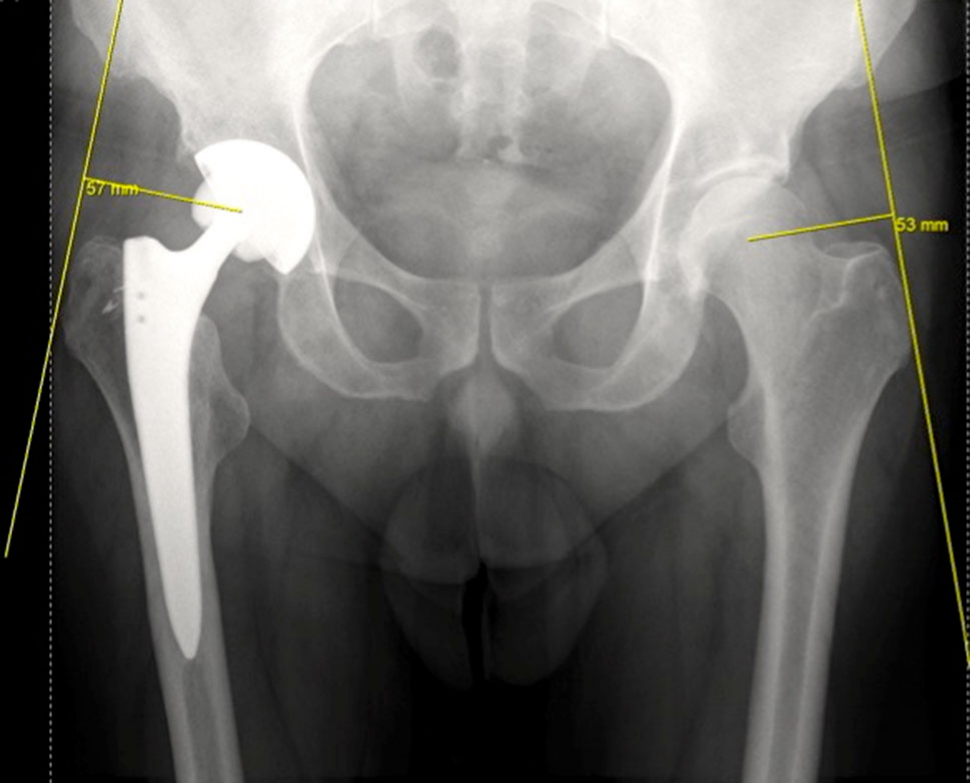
Radiograph demonstrating the abductor lever arm (ALA), defined as the distance from the center of rotation to the line of action of the abductor muscles
